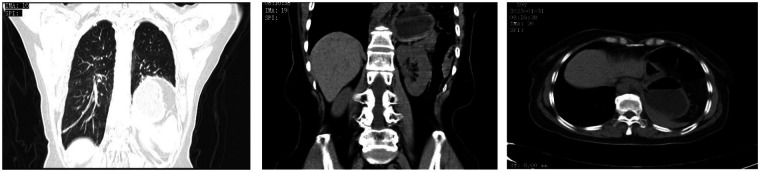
The CT scan showed left diaphragmatic eventration and a hiatal hernia.
